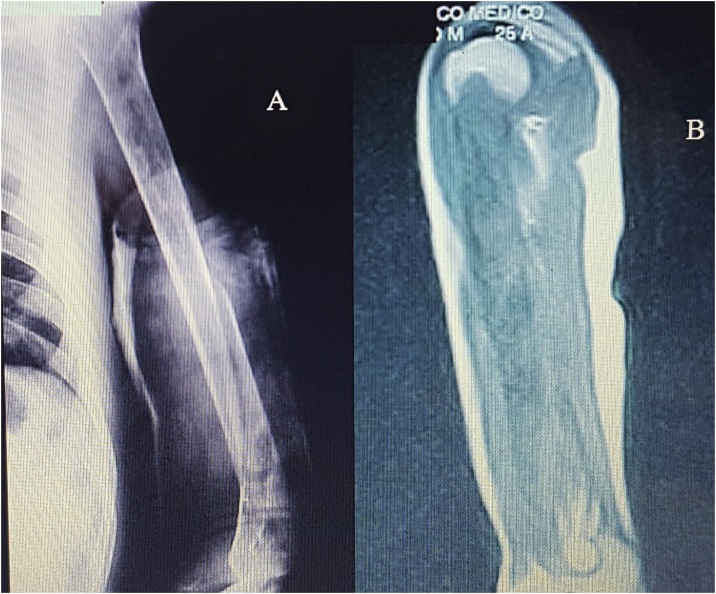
An AP x-rays showing humeral shaft fracture. B MRI showing total humerus compromise.
